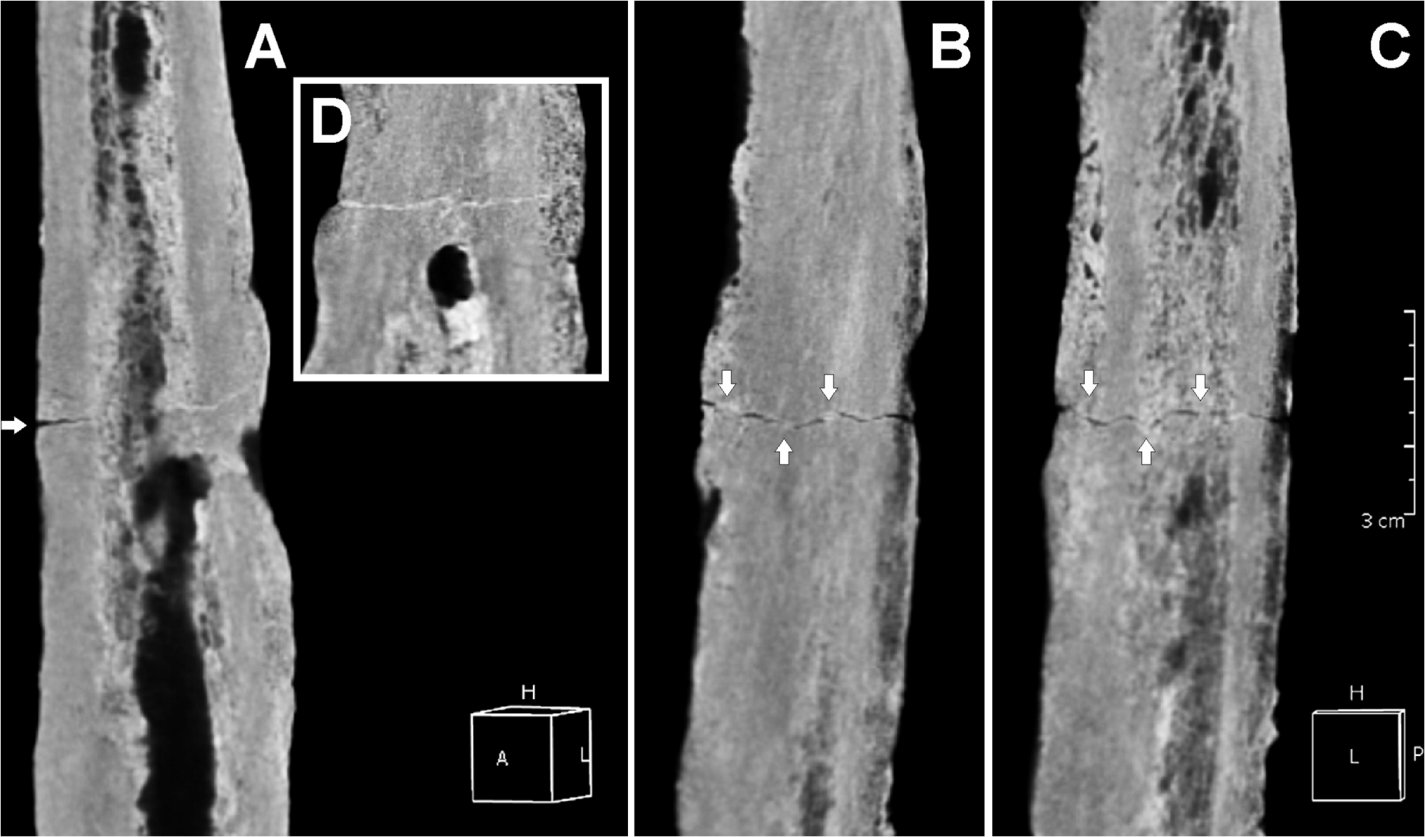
Three-dimensional (3D) computed tomography (CT) scan of the femoral lesion. Posterior (A), anterior (B), and medial (C) views of the transverse simple linear fracture. New bone apposition (*involucrum*) is evident. The bone lesion is characterized by poor healing (arrows) (D). Detail of the cloaca opening (box) and, above, the uninterrupted fracture line. Scale bar equals 3 cm.
